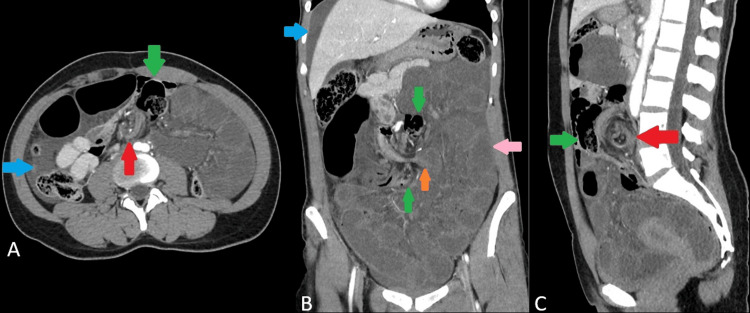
CT scan abdomen with contrast (axial, coronal and sagittal views) Contrast-enhanced CT abdomen axial view (A), coronal view (B), and sagittal view (C) showing twisting of the mesentery, giving a whirlpool pattern (red arrows in A and C), with resultant dilatation of the small bowel loops (orange arrow in B), which also show decreased enhancement, suggesting ischemia (pink arrow in B). Notice the sigmoid colon, which is also displaced superiorly and rotated around the mesenteric axis (green arrows in A, B, and C), and ascites (blue arrow in A and B). No pneumatosis, portal venous gas, or pneumoperitoneum is seen. CT: computed tomography
